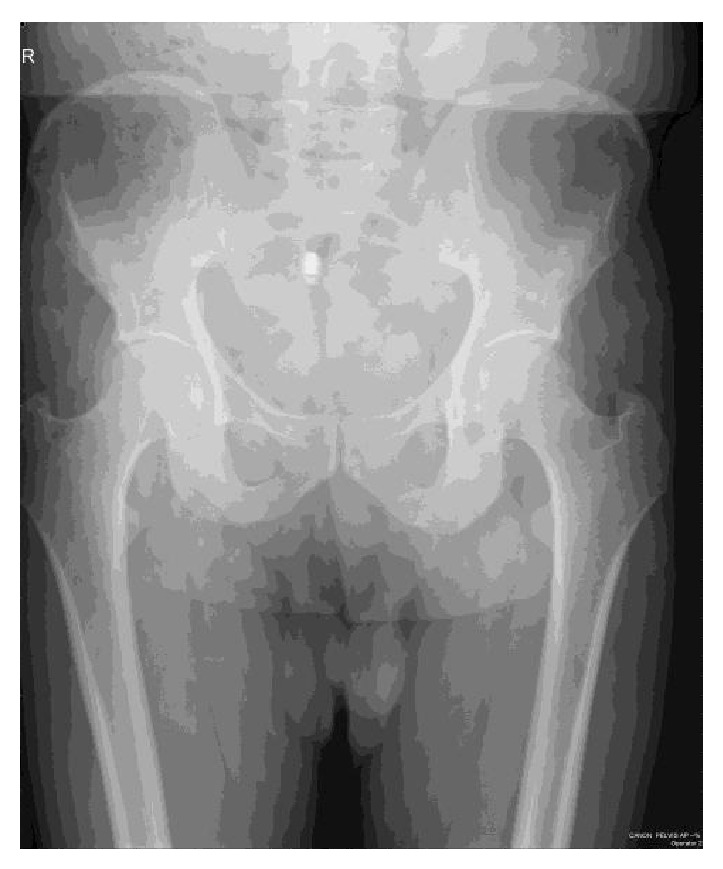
Anteroposterior plain radiograph of the pubis is normal.
